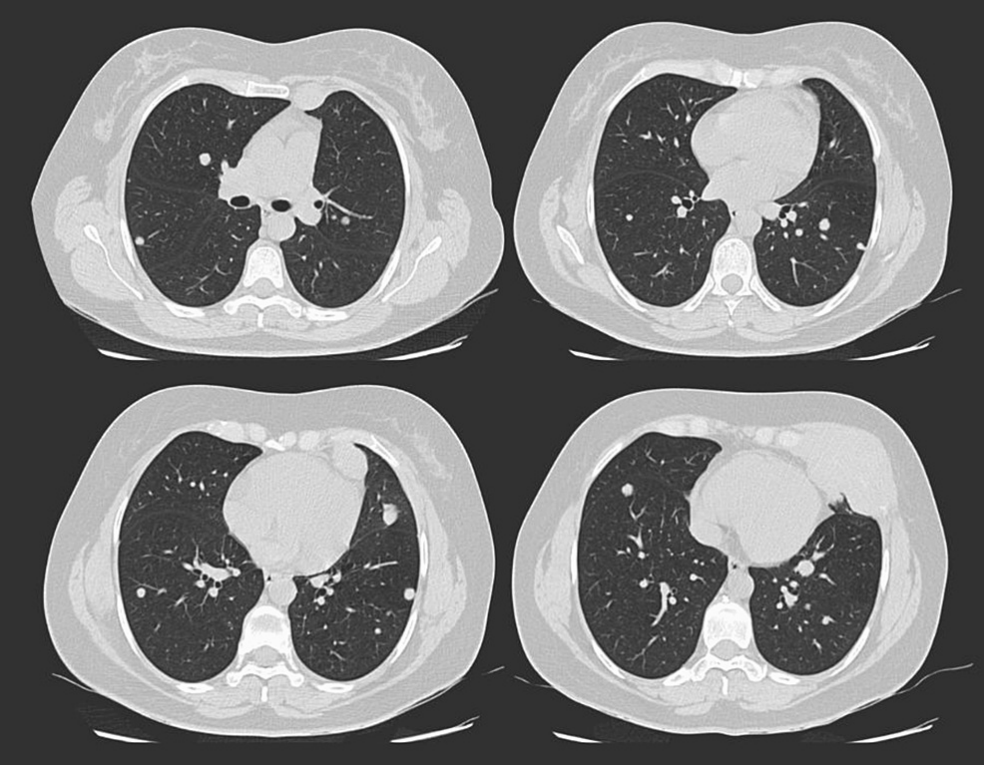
Multiple lung nodules seen in several axial slices in CT scan.
